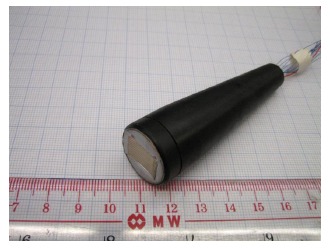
Photography of as-fabricated cylindrically shaped ultrasonic linear array.
